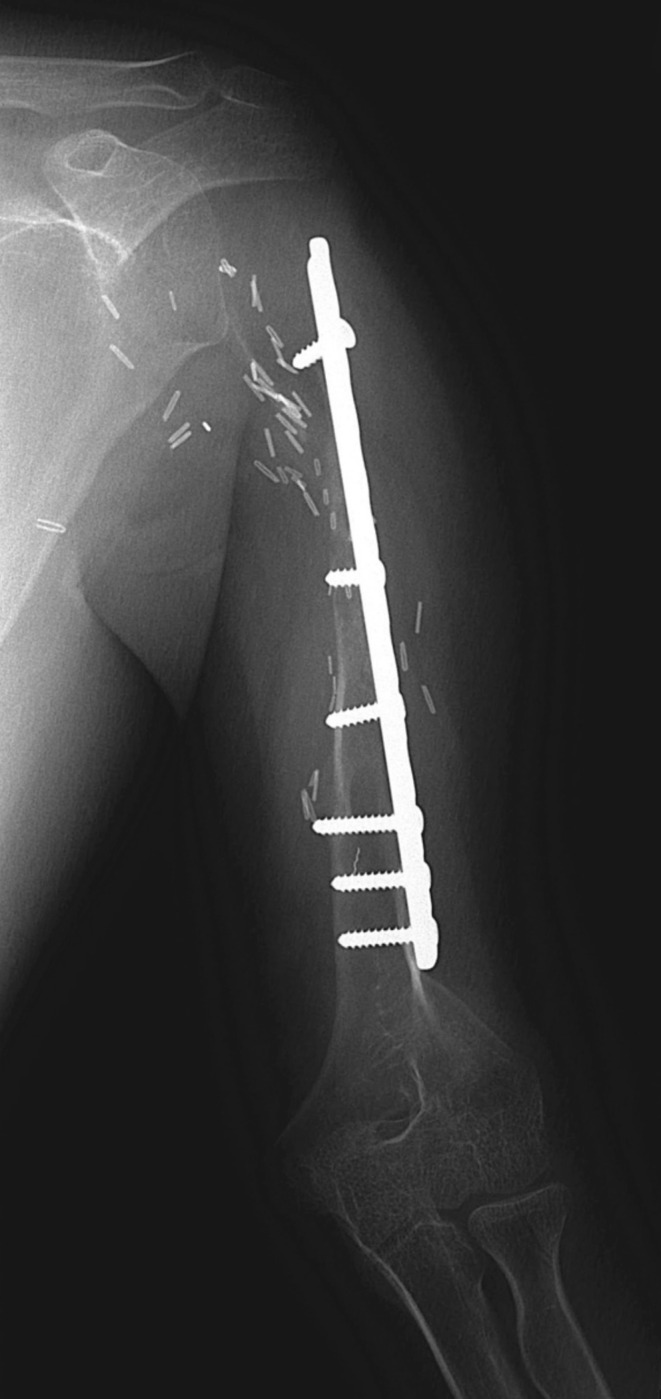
Plain radiograph of a paediatric patient who underwent fibular vascularized bone graft reconstruction following proximal humerus resection for Ewing's sarcoma. This was complicated by traumatic graft fracture and resorption.
